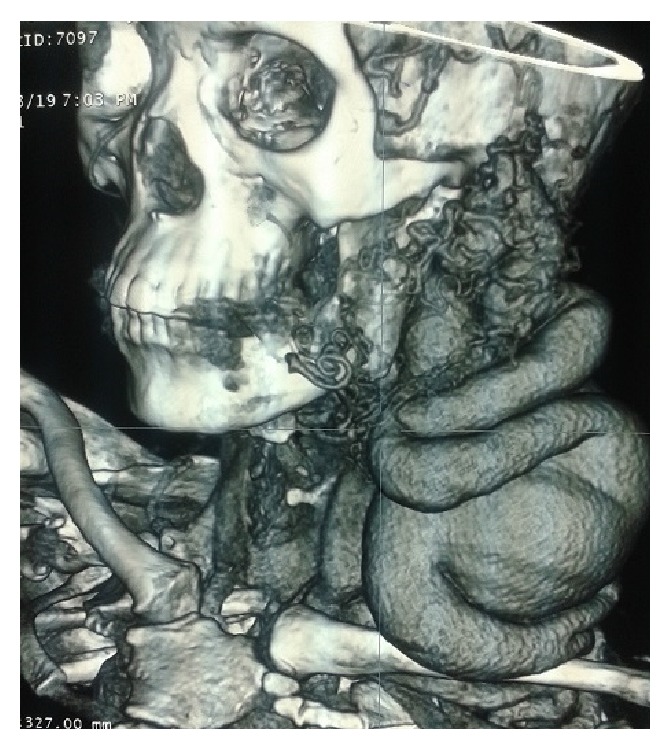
CT scan image of the cervical AVM. Anterolateral view showing connections with carotid, jugular, and subclavian.
